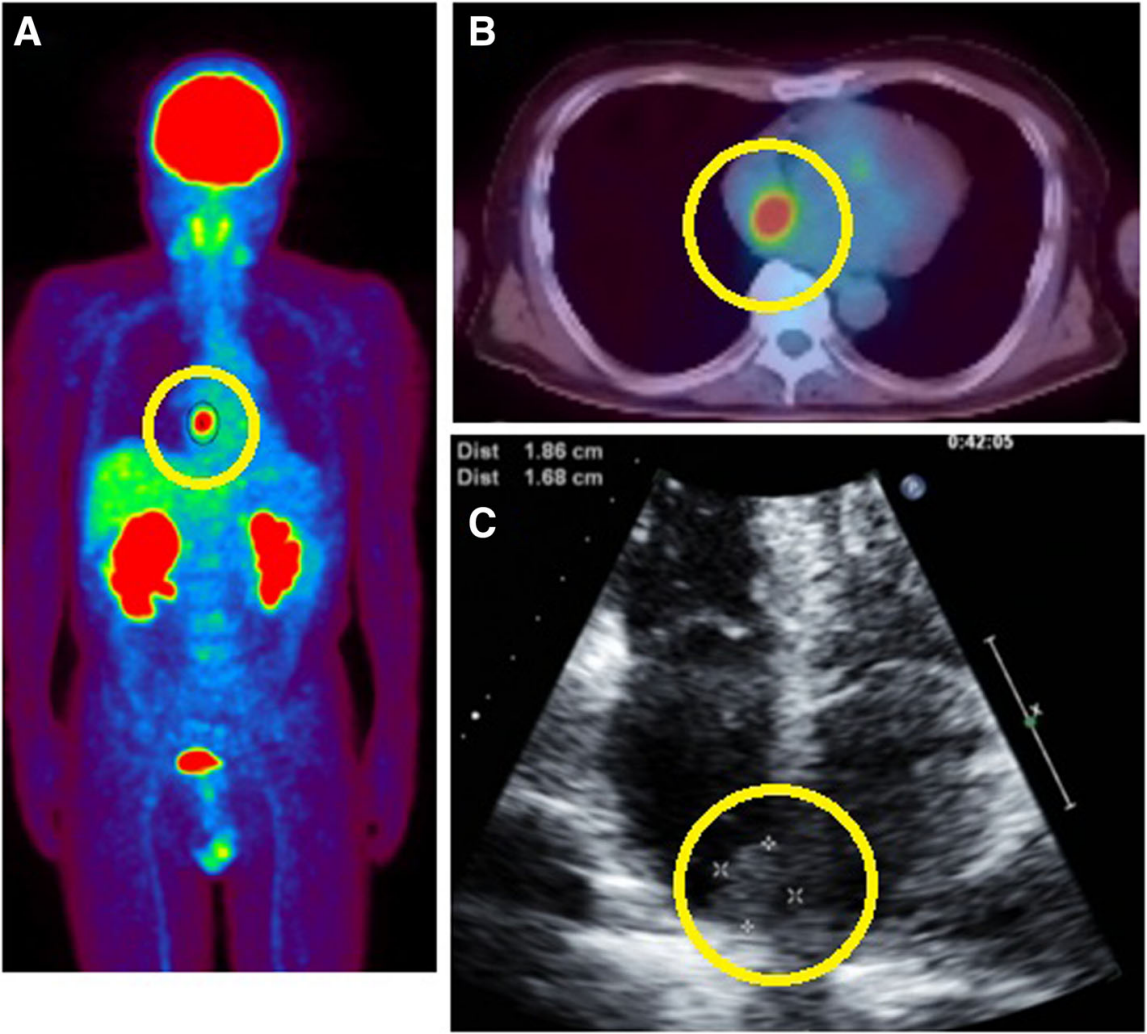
Positron emission tomography-computed tomography. a and b: Fluorodeoxyglucose uptake in the heart (*circle*), with a maximum standard uptake value (SUVmax) of 9.4. c: Echocardiography revealed a 16.8×18.6 mm ill-defined, interatrial septal mass (*circle*) protruding into the right atrium
